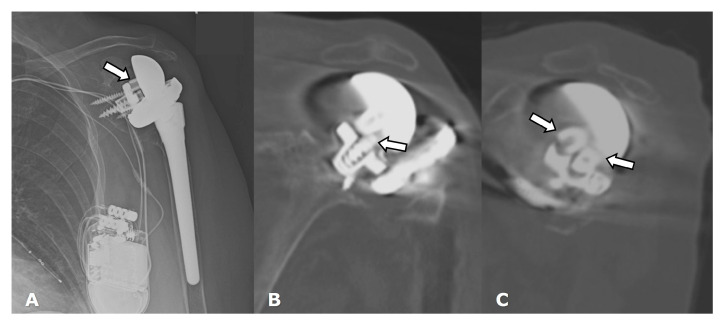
(A) Frontal radiograph and (B) coronal and (C) parasagittal CT images in a patient with RTSA show dissociation of the glenosphere from the metaglene/baseplate central screw (arrows).
